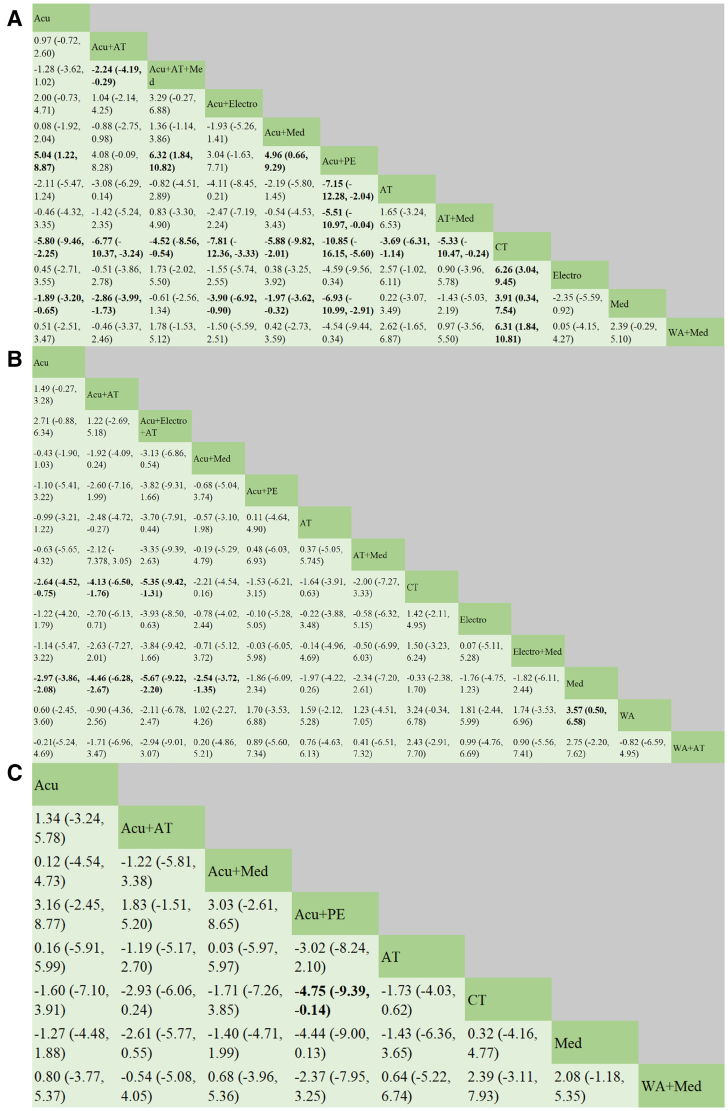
Network meta-analysis of the PSQI total score comparisons stratified by treatment duration. (A) < 4 weeks; (B) 4 weeks; and (C) >4 weeks. Acu = acupuncture, AT = auriculotherapy, CT = conventional treatment, Electro = electroacupuncture, Med = western medicine, PE = point embedding, PSQI = Pittsburgh Sleep Quality Index, WA = warm acupuncture.
